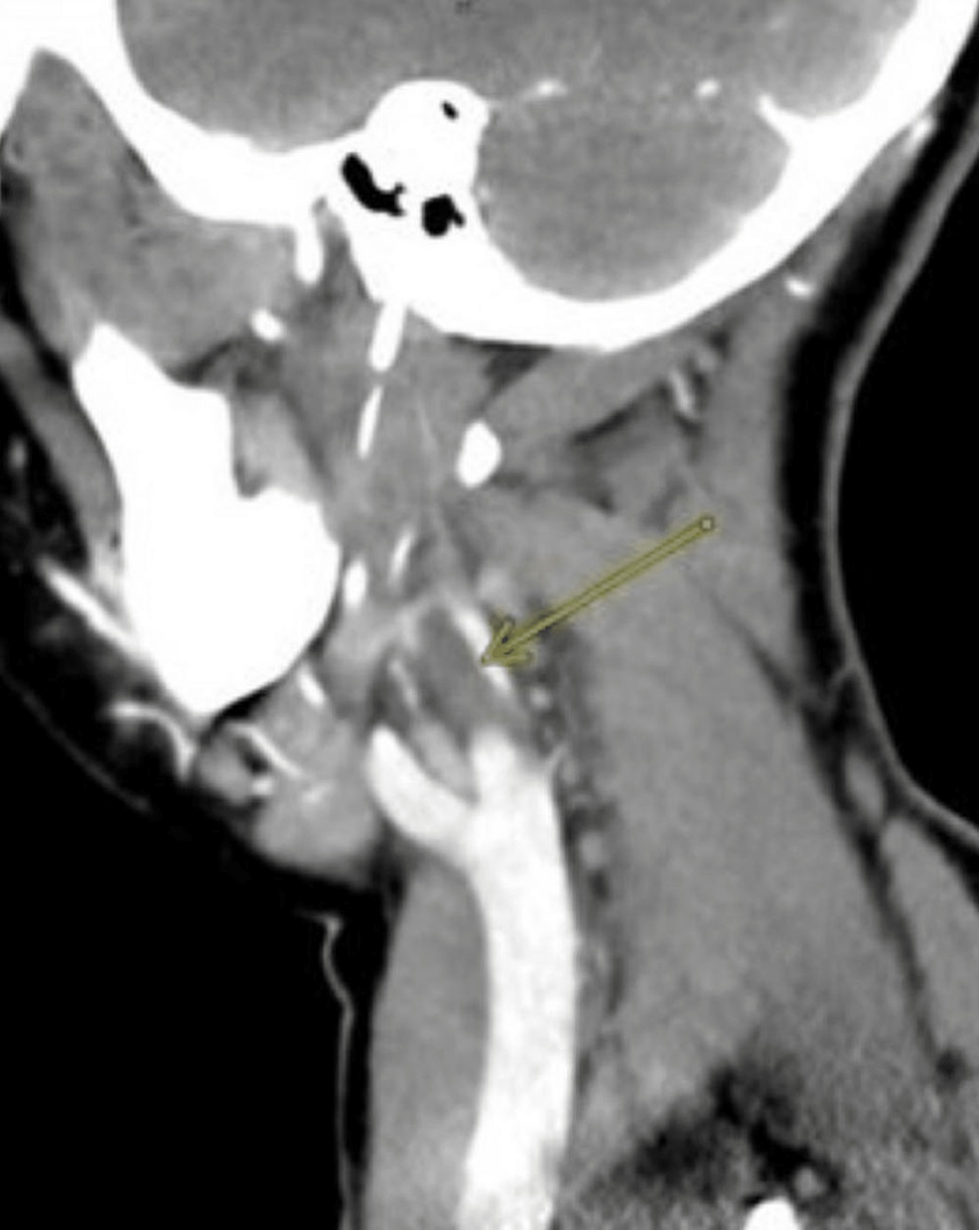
Sagittal view of CT with contrast demonstrating thrombus in the right IJV (yellow arrow). IJV, internal jugular vein
